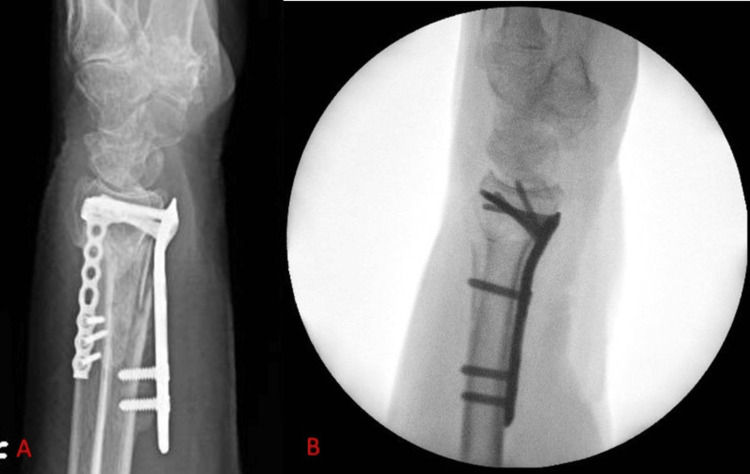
Lateral radiographs from two different cases, showcasing the importance of adequate shaft fixation in osteoporotic bone. (A) Lateral postoperative radiograph, demonstrating loss of fixation of the volar locking plate on the distal radius. Note that the plate is fixed to the shaft with only two screws. (B) Lateral postoperative radiograph demonstrating appropriate shaft fixation with three locking screws. Note that the non-locking slot has been left empty as the non-locking screw did not fully engage the far cortex due to comminution. Despite this, the three remaining locking slots on the plate allowed for adequate fixation to the shaft.
